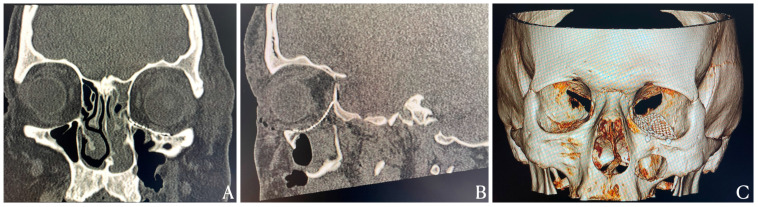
Postoperative imaging confirmed successful reconstruction of the orbital floor using a titanium mesh implant. As shown in Figure 3 coronal CT scans revealed (A) symmetrical restoration of orbital floor continuity, with partial reconstruction of the medial wall. Sagittal views showed re-establishment of orbital floor integrity extending posteriorly to the bony ledge (B). Three-dimensional CT reconstructions provided a comprehensive view of the restored orbital anatomy, confirming accurate implant alignment and positioning (C). The primary objectives of orbital floor reconstruction are to restore the integrity of the orbital walls, re-establish ocular motility, and prevent delayed enophthalmos, thereby optimizing both functional and aesthetic outcomes. A wide range of materials can be utilized for orbital reconstruction, including autogenous grafts harvested from the anterior wall of the maxillary sinus, iliac crest, or auricular and nasal septal cartilage. Among alloplastic materials, titanium mesh remains the most commonly employed non-resorbable implant due to its excellent biocompatibility, high mechanical stability, and intraoperative malleability, which allow for precise replication of the native orbital contour [9]. A recent systematic review and meta-analysis by Kotecha et al. reported a trend toward improved postoperative orbital volume restoration, reduced enophthalmos, and shorter operative times with the use of patient-specific implants (PSIs) compared with conventional techniques. However, these differences did not reach statistical significance, suggesting that current evidence does not yet confirm the superiority of PSIs. Consequently, the selection of the reconstructive material should remain at the surgeon’s discretion, guided by clinical context and intraoperative findings [10].
